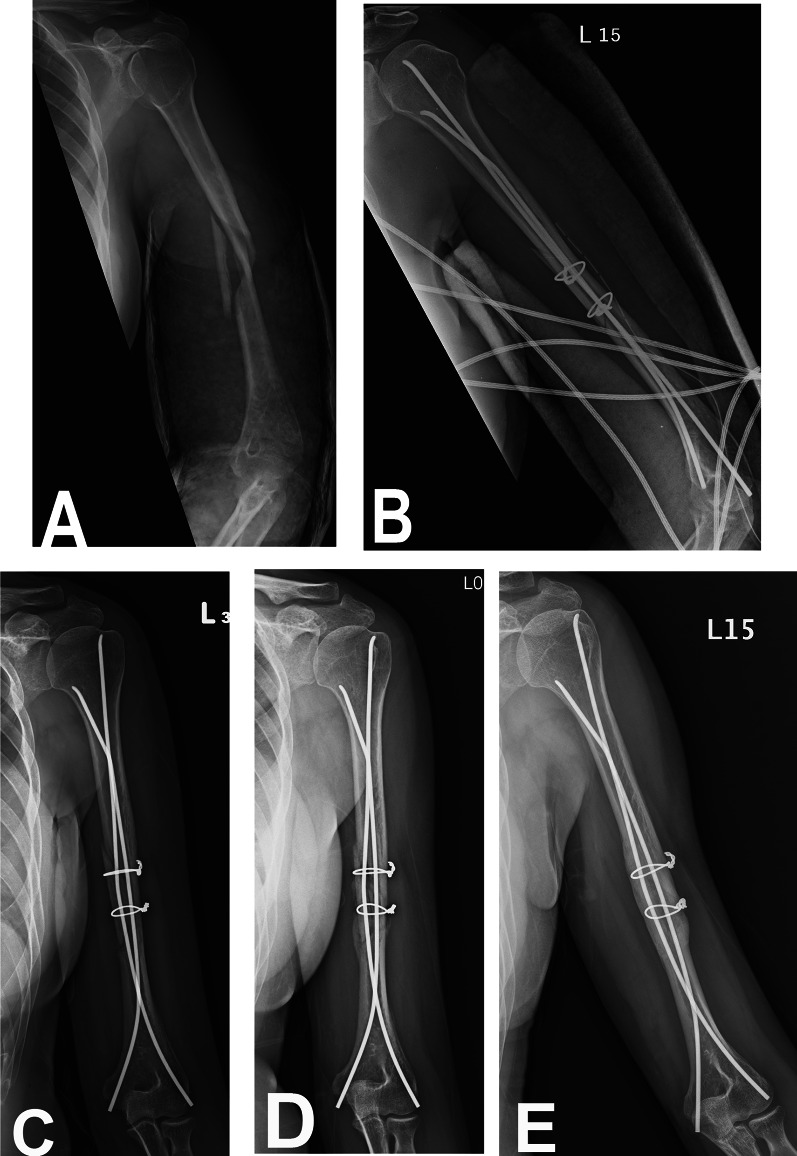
Case demonstration of a 61-year-old female patient: a preoperative anteroposterior radiograph of the humerus; b postoperative radiograph; c postoperative radiograph at 4 weeks’ follow-up; d postoperative radiograph at 8 weeks’ follow-up, showing that the bridging callus and fracture line were diminished; e postoperative radiograph at 6 months’ follow-up, showing solid union
